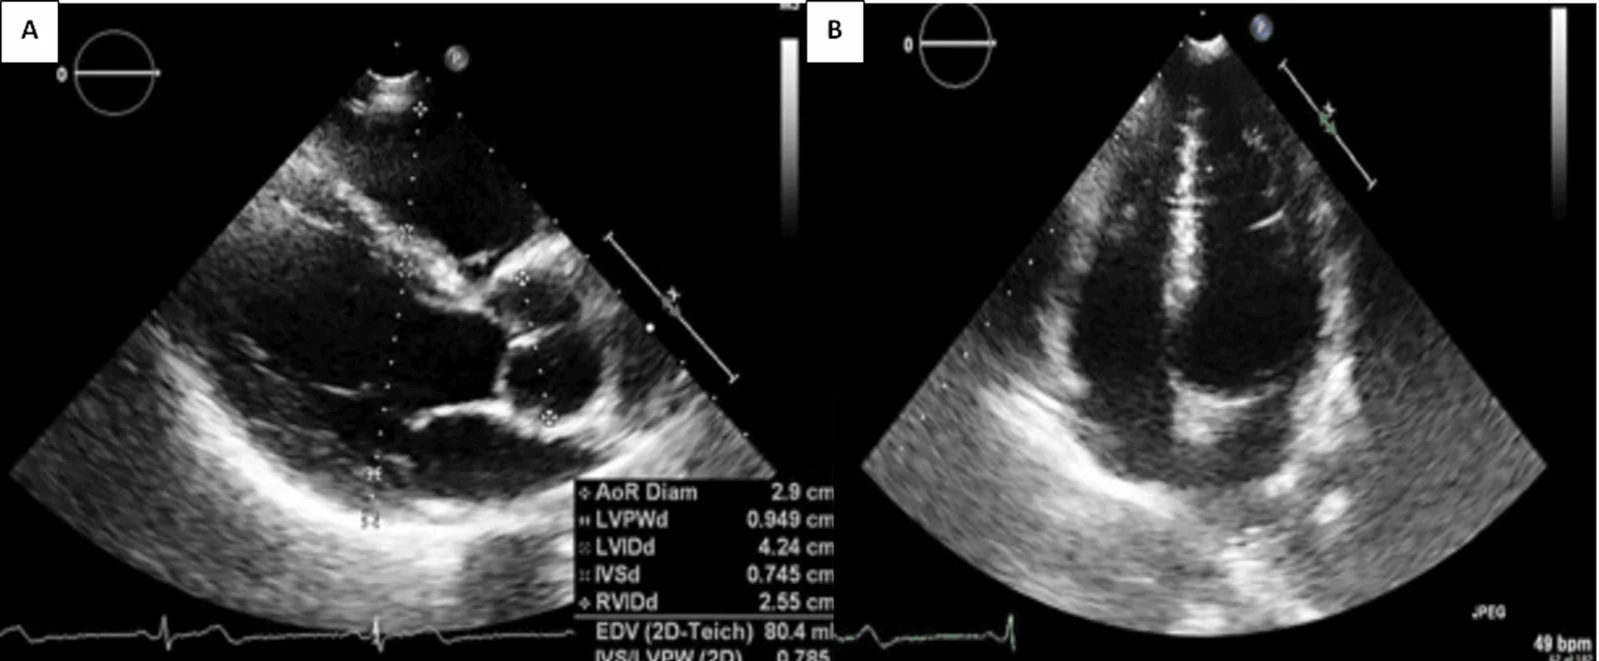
Two-dimensional transthoracic echocardiogram. (A) Parasternal Long-Axis View: Demonstrates normal left ventricular (LV) structure and systolic function. No pericardial effusion or signs of cardiac compression/tamponade are observed. (B) Apical 4-Chamber View: Confirms preserved left ventricular ejection fraction (LVEF). No intracavitary masses, thrombi, or tumors are visible in any chamber.
